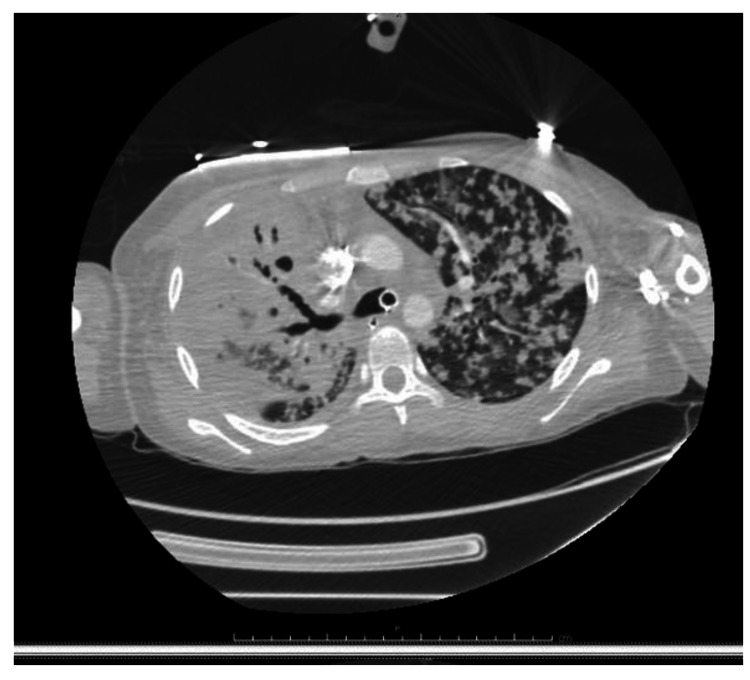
Computed tomography angiography (CTA): Extensive pulmonary opacities
